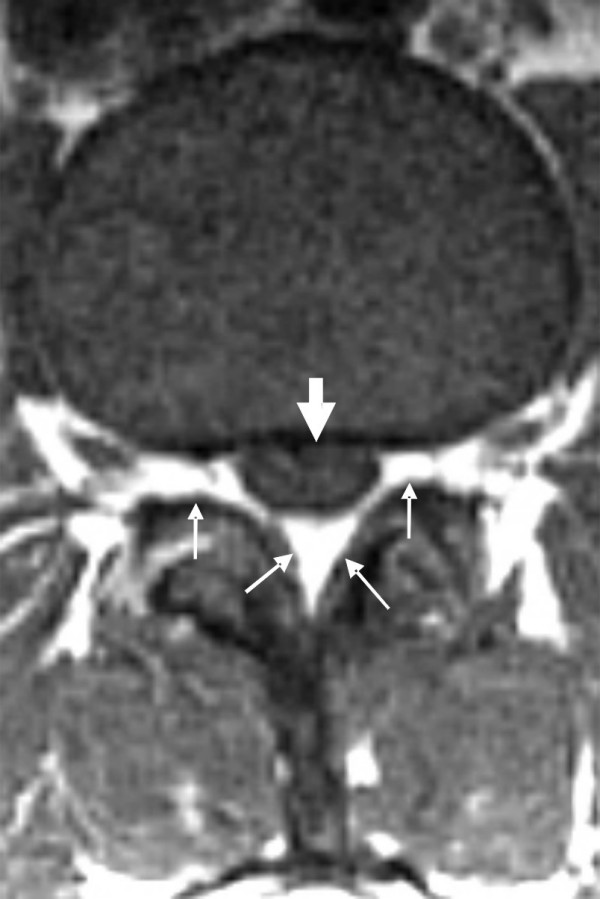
A T1-weighted cross-sectional magnetic resonance image at the level of lumbar puncture (T2-weighted cross-sectional images were not obtained). The bony spinal canal is congenitally relatively small. The cross-sectional area of our patient's dural sac was 80 mm^2^. With excess epidural fat of LEL 2 according to Borré *(small arrows)*, the dural sac completely outlines the bundle of nerve roots (greyish appearing, *large arrow*) leaving no free cerebrospinal fluid space, which would have appeared nearly black. These nerve roots are not completely free to move away when a needle is inserted into the dural sac.
